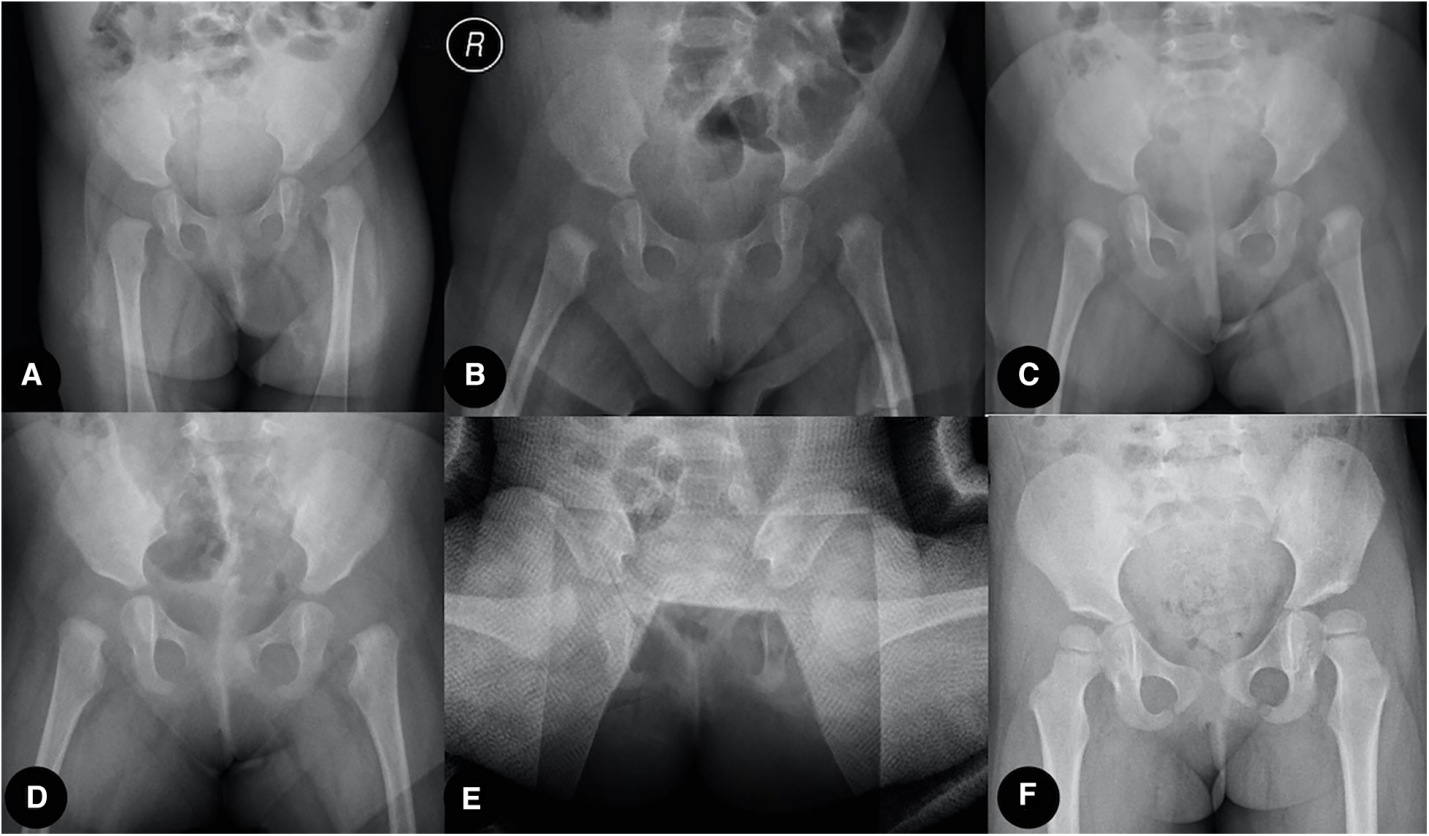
Two-stage traction reduction group case: (A) Five-month-old girl patient with left hip dislocation. (B–D) At-home horizontal traction achieved a sufficient proximal femoral physis descent, and the ultrasound-guided in-hospital vertical traction was applied. (E) After being evaluated by arthrography, the hip was immobilized in a spica cast for 3 months. (F) Reduction was maintained at the final follow-up.
